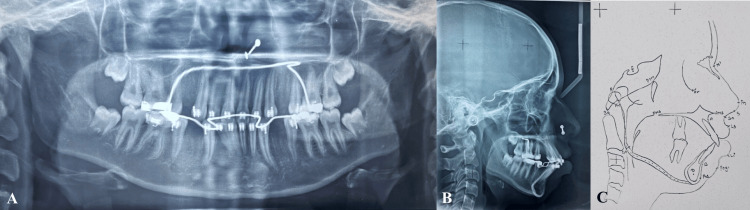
Stage radiographs: (A) Orthopantomogram; (B) lateral cephalogram; (C) tracing of lateral cephalogram.
